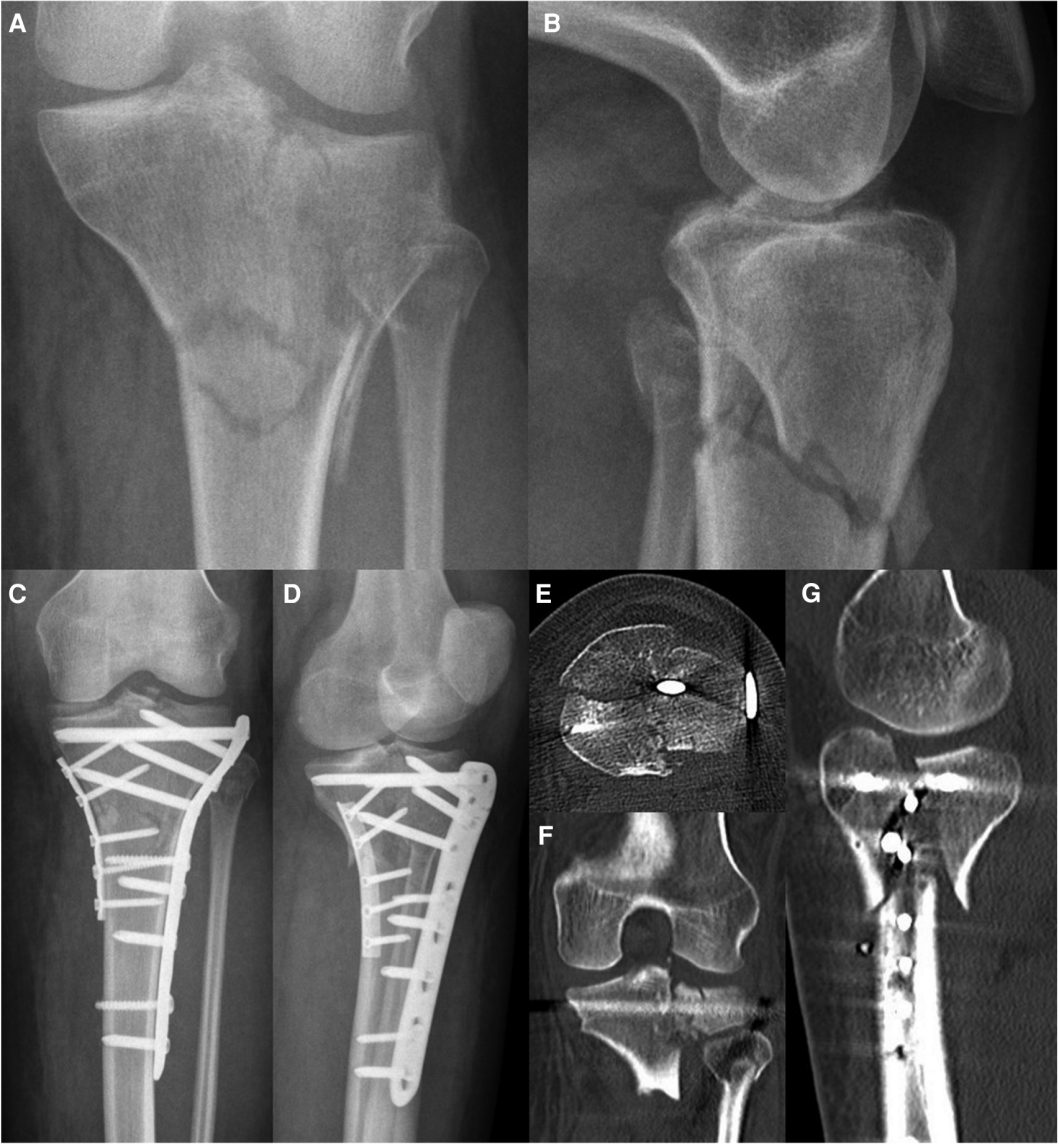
Case 5, 27 year female patient, motorcycle accident during holidays. 3-column fracture (Schatzker VI, AO 41-C3, Moore V, 3-column-fracture) displayed by AP (A) and lateral (B) x-ray. Postoperative AP (C) and lateral (D) x-ray as well as axial CT cut (E), coronal (F) and sagittal (G) reconstruction after first surgery abroad (ORIF by double plate fixation *via* anterolateral and medial approaches) reveal a persisting dislocation of a large posteromedial fragment causing an articular step-off of more than 5 mm.
